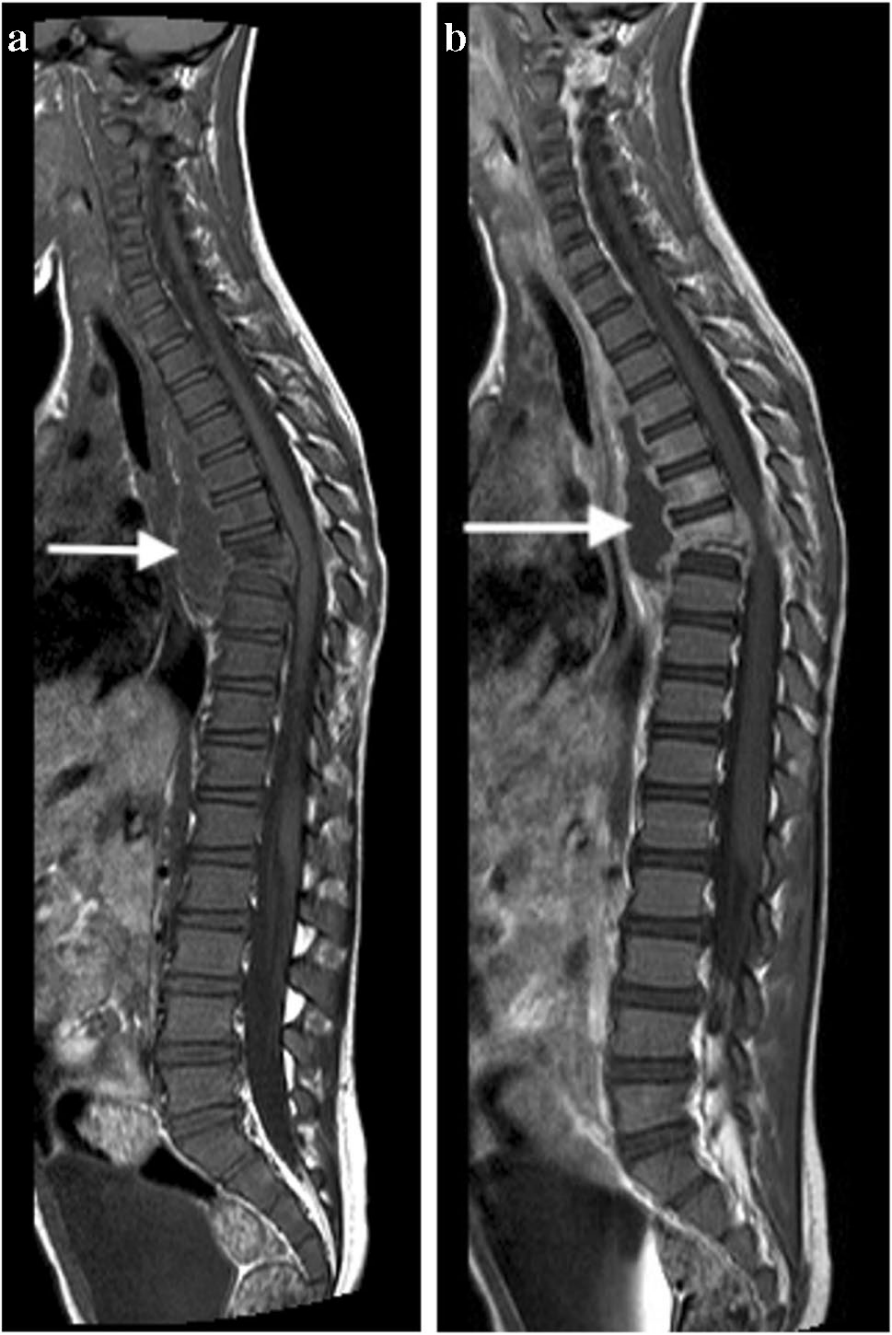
Sagittal T1 pre- (a) and post-gadolinium (b) magnetic resonance images in a 5-year-old girl who presented with kyphosis. There is a hypointense peripherally enhancing anterior subligamentous collection (*arrows*) causing scalloping of the anterior vertebral bodies. There is kyphosis centred around the destroyed T7 and T8 vertebral bodies (identified by way of their intact posterior elements). Tuberculosis was confirmed
